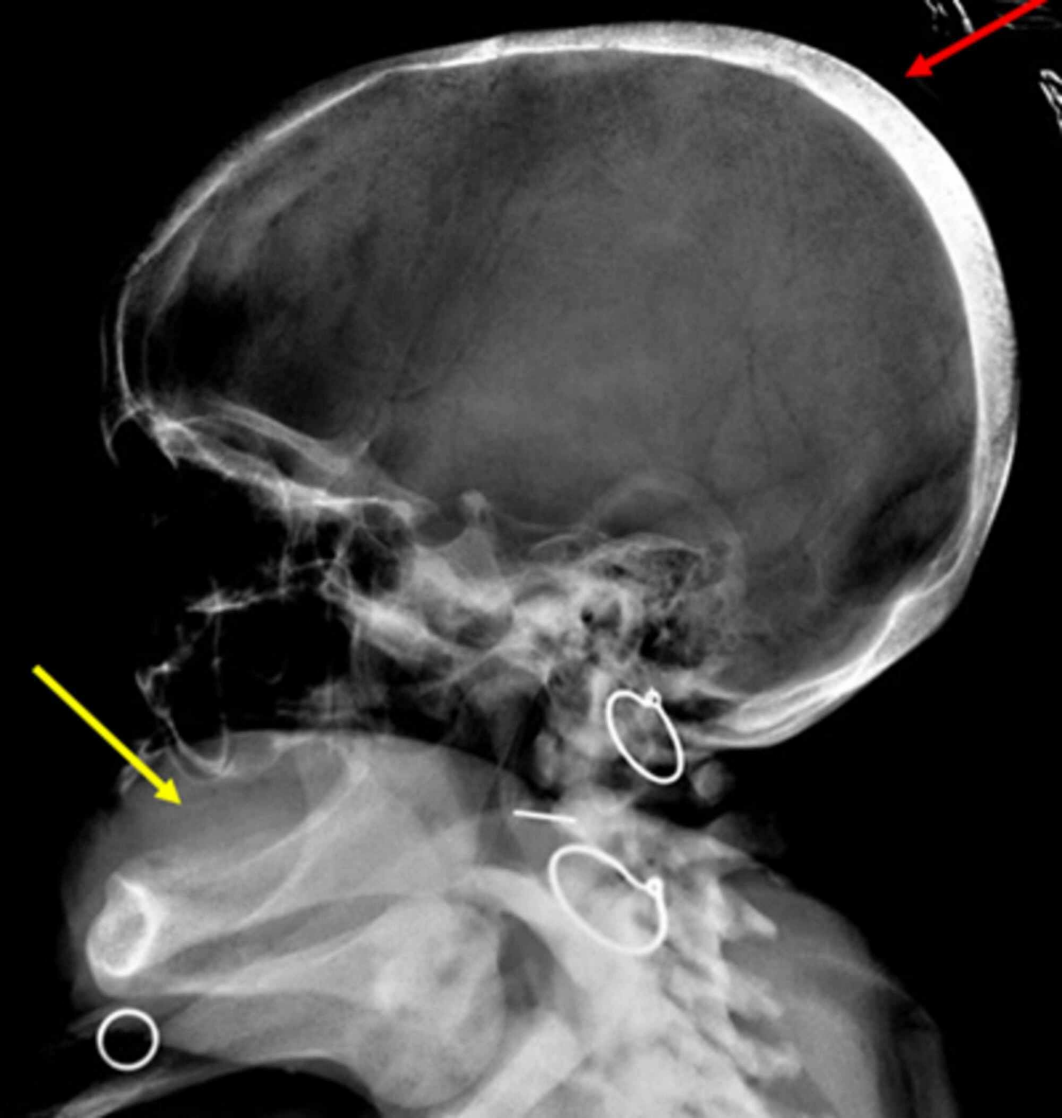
Skull radiograph The image shows cortical thickening at parieto-occipital calvaria (red arrow) and edentulous mandible, resorption of lamina dura with cortical thinning (yellow arrow)
